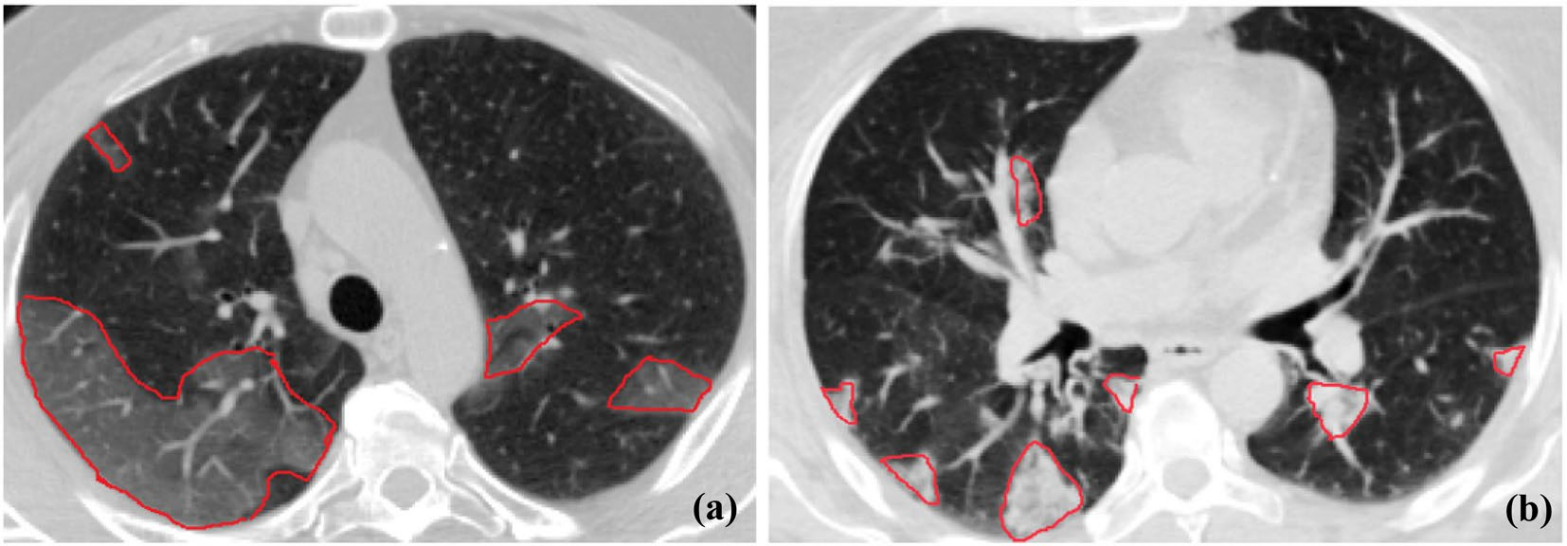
The most commonly observed CT patterns in COVID-19 pneumonia: (a) GGO pattern, and; (b) Consolidation pattern. Infection regions have been identified with red borders^13^.
